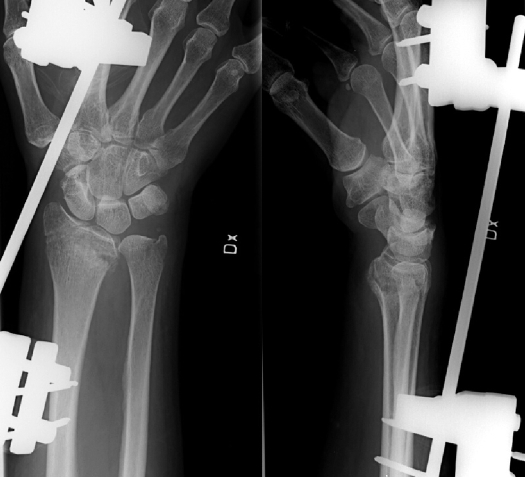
AP and lateral radiographs of a patient operated using closed reduction and external fixation.
